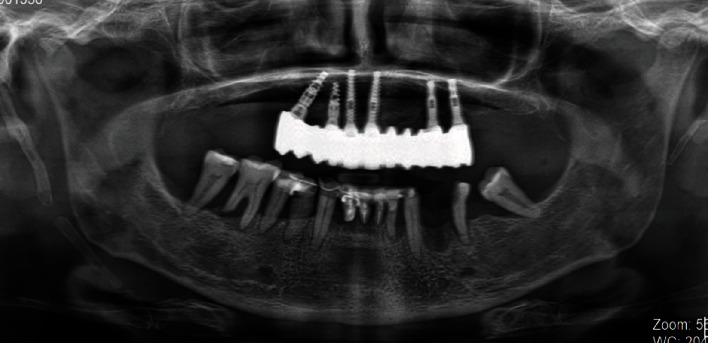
Orthopantomography performed in 2021.
